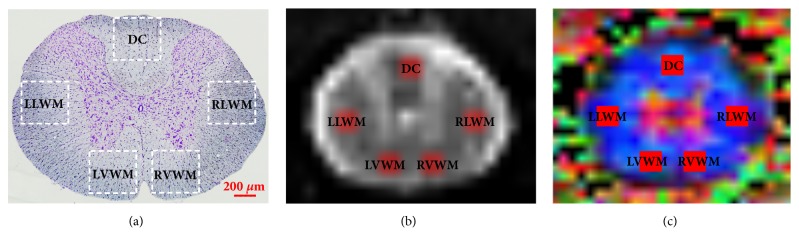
Set of different tractography ROI regions. (a) Pathological image. (b) MRI image. (c) Color FA map. DC (dorsal column), LLWM (left lateral white matter), RLWM (right lateral white matter), LVWM (left lateral white matter), and RVWM (right lateral white matter).
